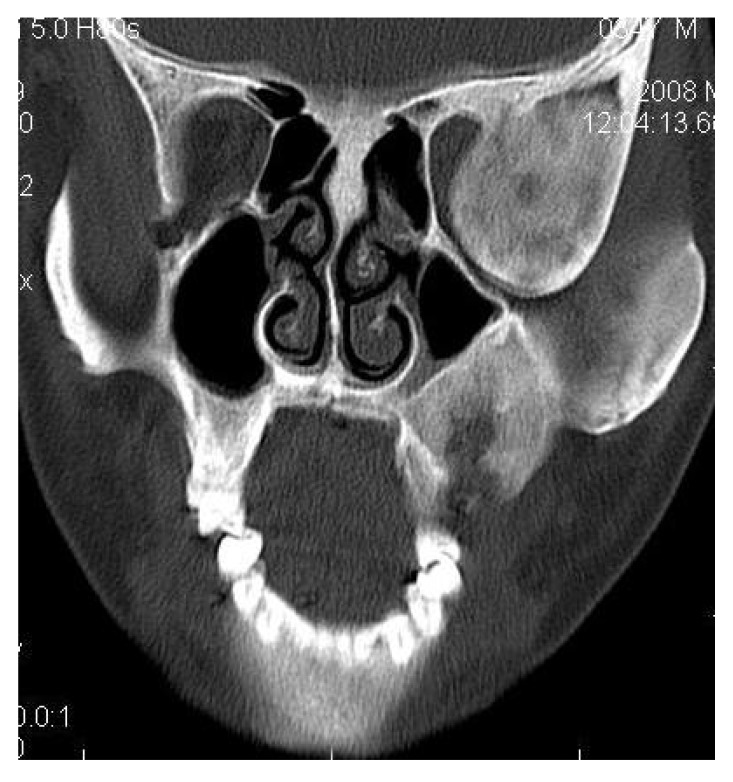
The coronal CT section revealing the involvement of the right orbit, maxilla and right Zygomatic arch and right posterior maxillary alveolar process.
